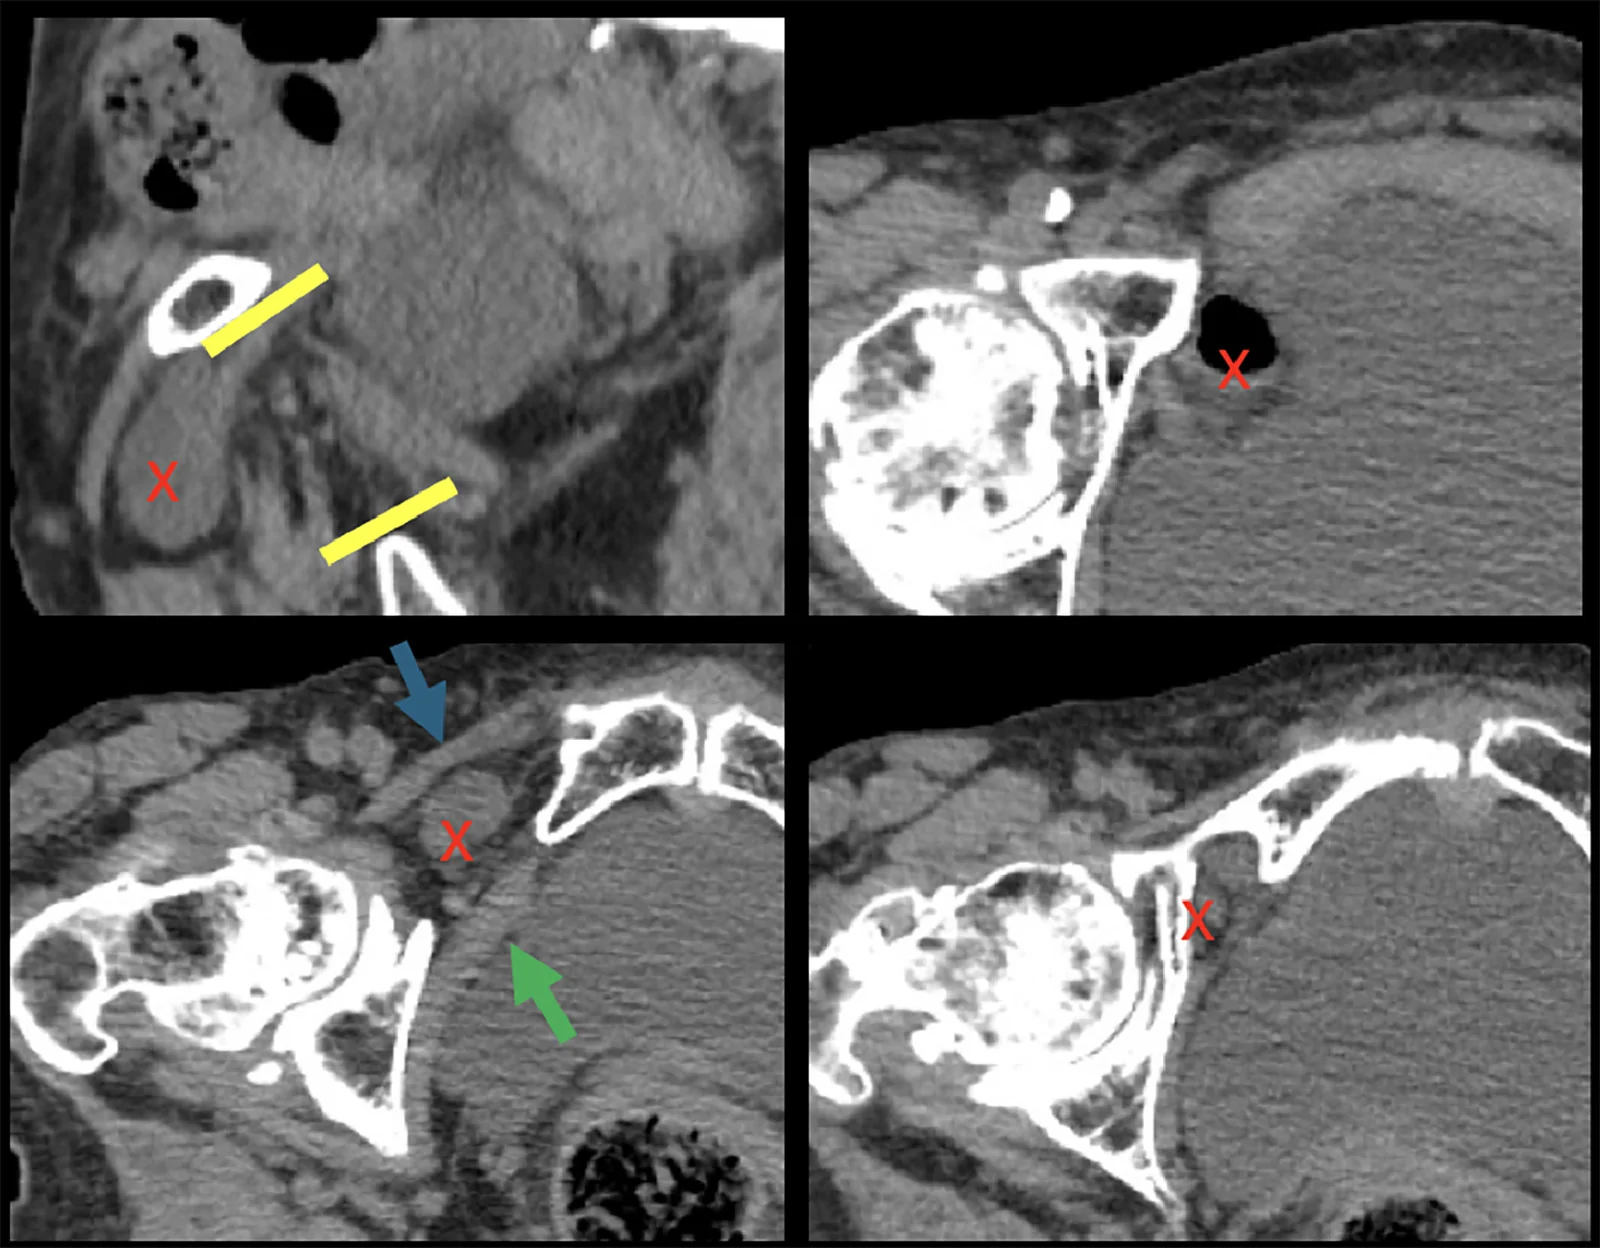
Clockwise from top left. Sagittal noncontrast CT image demonstrates a loop of bowel (red X) herniating through the right obturator foramen (yellow lines). Sequential axial CT images demonstrate a loop of bowel extending from right hemipelvis to the right obturator canal between the pectineus (blue arrow) and obturator (green arrow) muscles. The obturator canal is widened and measures approximately 2.2 cm. The left obturator canal is unremarkable (not shown).
